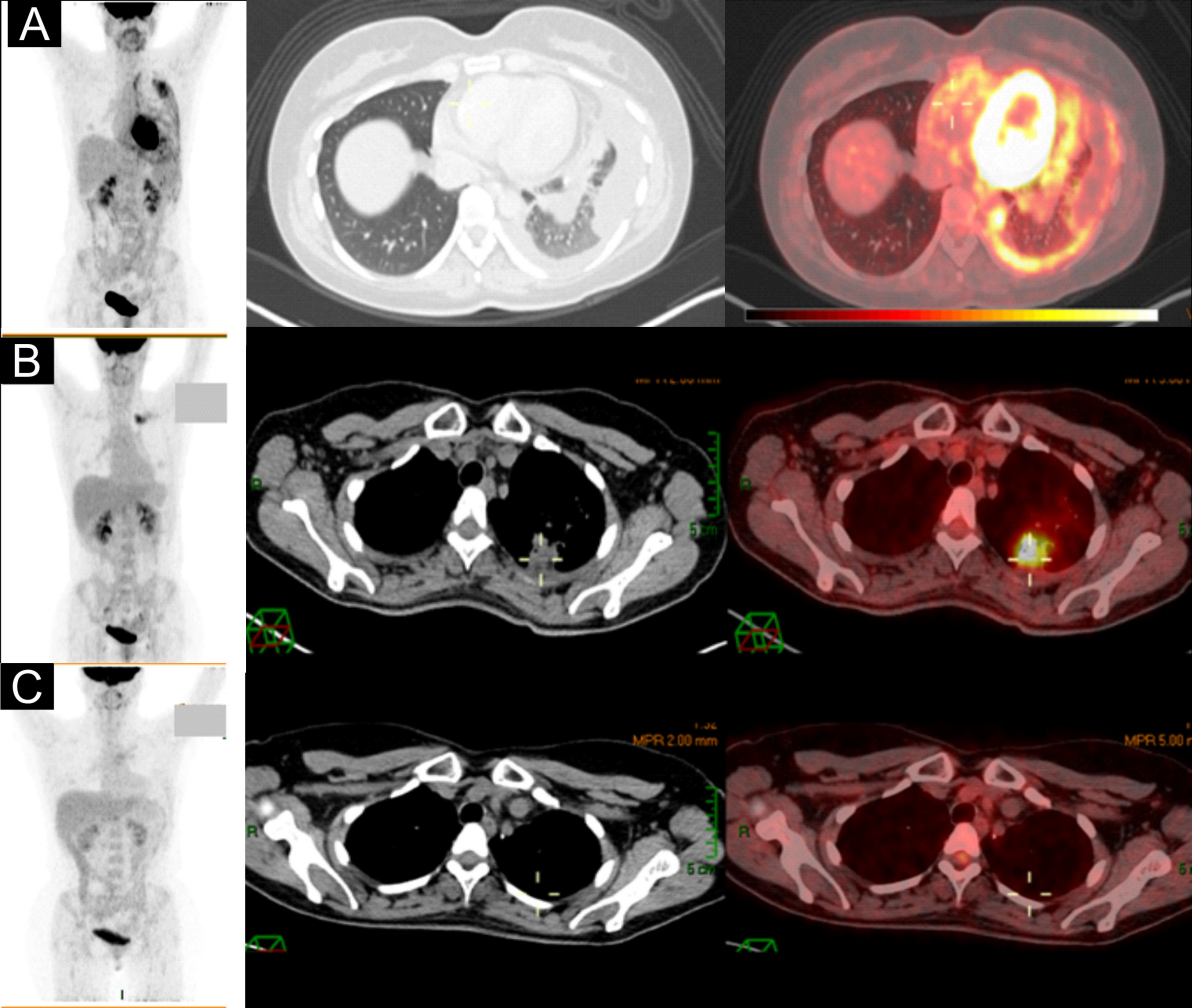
(A) Baseline PET-CT showed nodular thickening of the left parietal pleura, lung lesion in the left upper segment, and loculated pleural effusion. (B) PET-CT after SBRT revealed an increase in size and 18-FDG uptake. (C) PET-CT after 34 months of afatinib with complete response.
